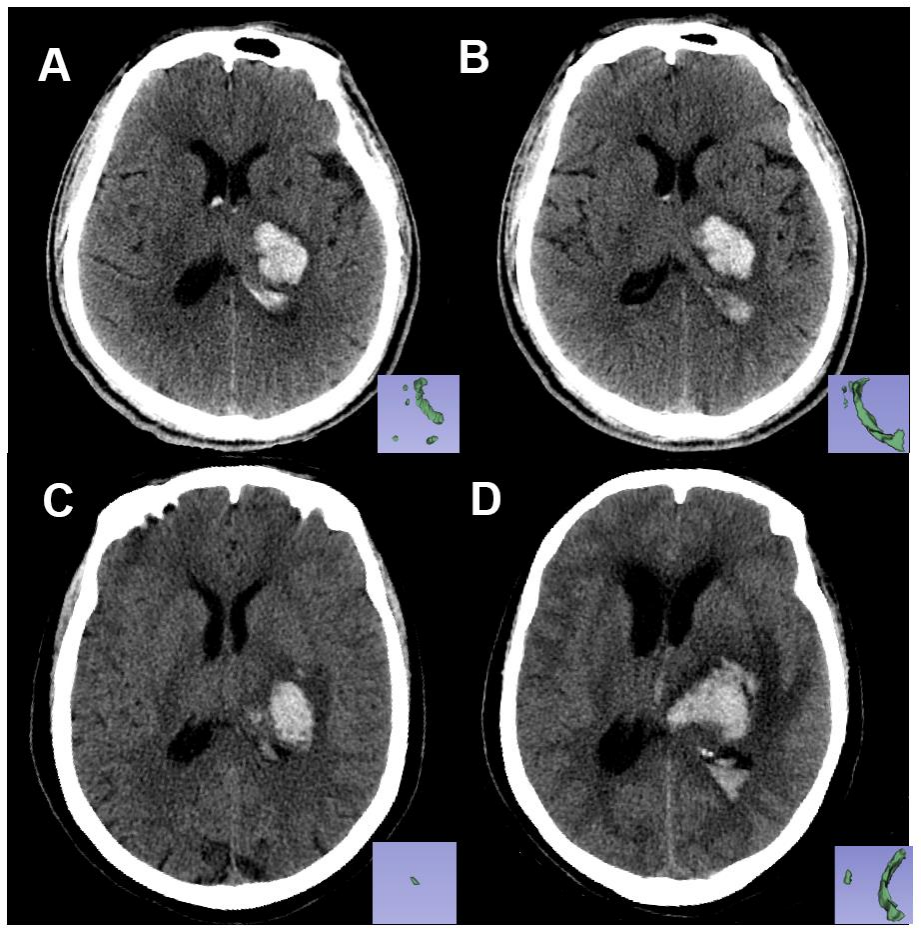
Non-contrast CT images of two patients with similar hematoma but different experiences. Pictures in the lower right corner are the 3-D images of the IVH. Image (A, B) are the baseline and follow-up CT images, respectively, of patient A: a 61-year-old male who had a Rad-score of -2.2119681 (<-1.7259179). Image (C, D) are the baseline and follow-up CT images, respectively, of patient B: a 67-year-old female with a Rad-score of -1.6176548 (>-1.7259179). Within 24 hours from symptom onset, the IVH volume of patient A changed from 5.62 mL to 6.84 mL, and the IVH volume of patient B changed from 0.5 mL to 12.4 mL. Patient B experienced IVH growth while patient A did not.
